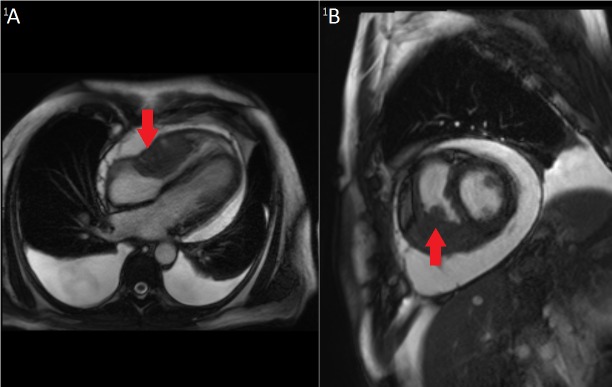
Cardiac MRI of the Tumor A: Axial T2-weighted and B: Sagittal T2-weighted MRI images showing large tumor (arrows) in the right ventricle obstructing flow through the tricuspid valve and extending into the right atrium. Additional findings included a pericardial effusion and bilateral pleural effusions.
